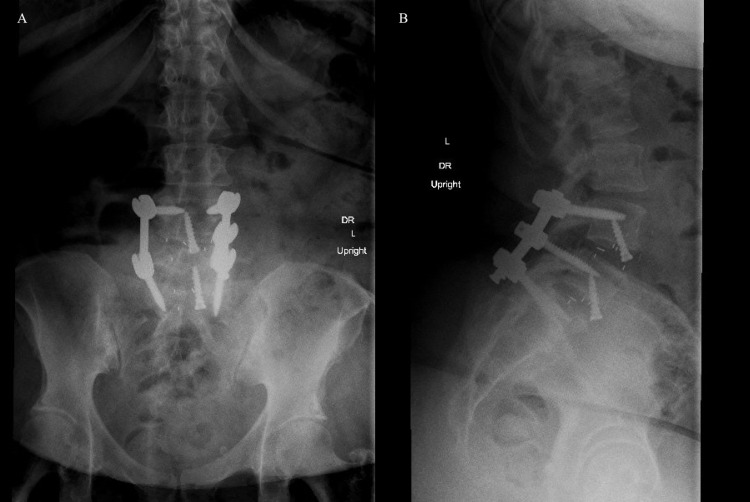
Postoperative upright AP (A) and lateral (B) x-rays AP - anteroposterior
